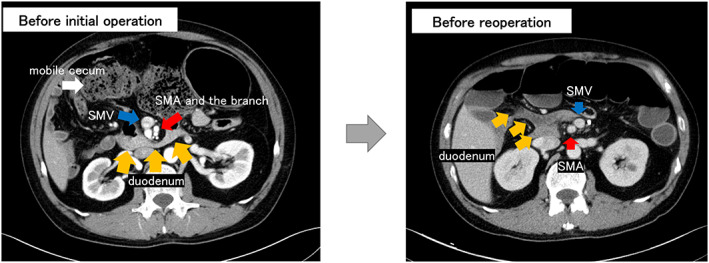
Images of computed tomography scan before initial operation and before reoperation. Before initial operation: the patient had a mobile cecum, and the third portion of the duodenum ran dorsal to the superior mesenteric artery (SMA) and superior mesenteric vein (SMV). Before reoperation: the duodeno‐jejunal transition was located on the right side of the abdomen, and the SMV was located to the left of the SMA
